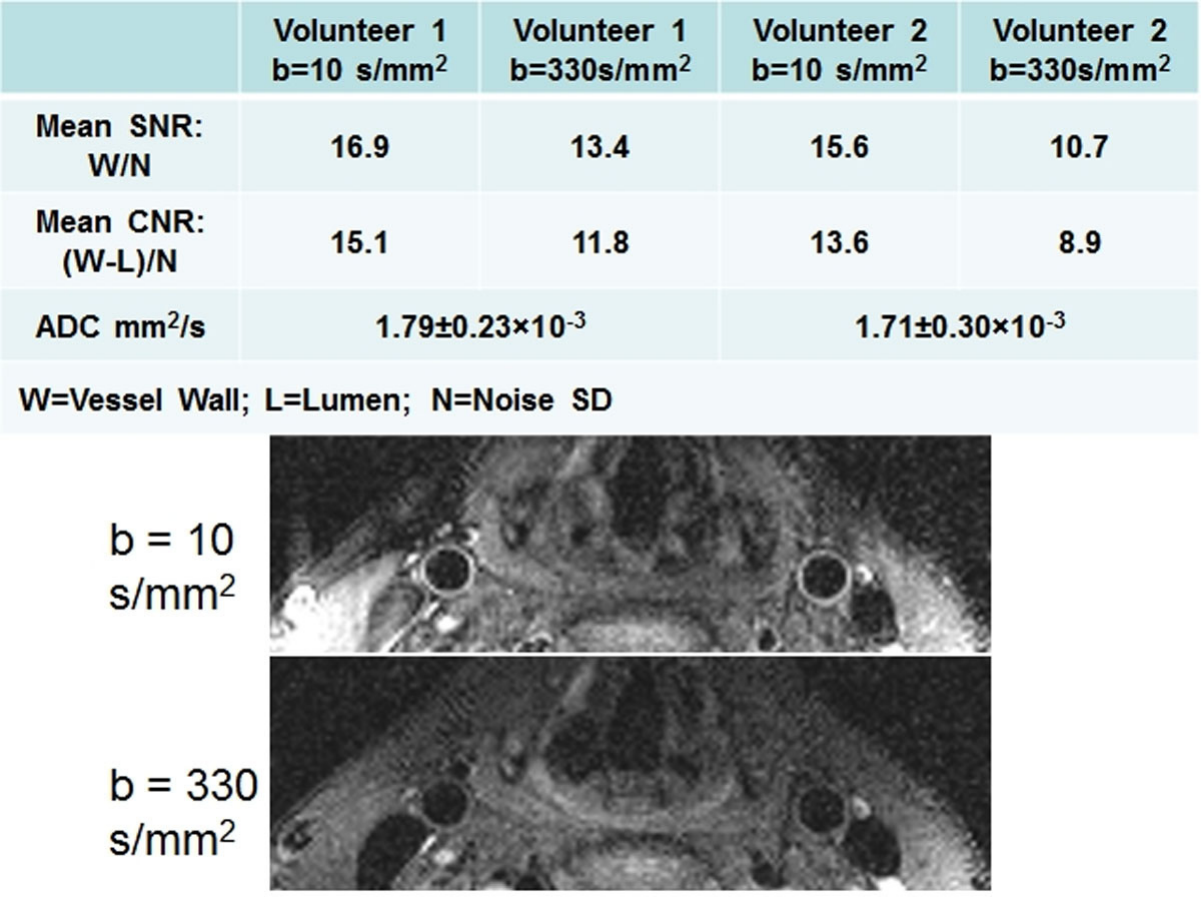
Top panel: mean relative SNR, CNR and vessel wall ADC values for two normal volunteers; Bottom panel: 3D TSE DWI of normal carotid vessel wall at two b values.
